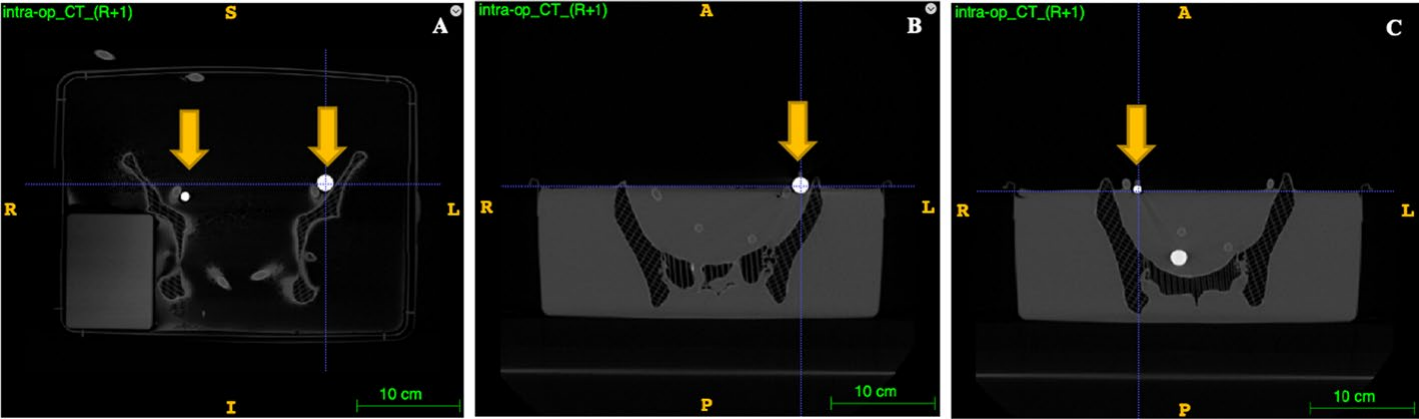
Computed tomography of the model. Coronal (A) and axial CT images showing the target SLNs in both sides (arrows: right SLN (B) and left SLN (C)) in arterial phase. CT, computed tomography; SLN, sentinel lymph node
